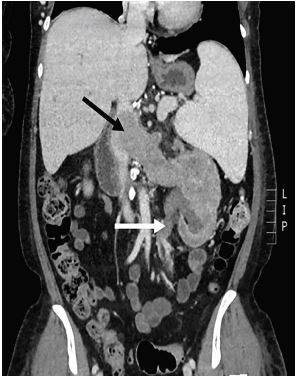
Coronal view of Computed Tomography of abdomen/pelvis with intravenous contrast demonstrating a necrotic mass in the left kidney with extension into the inferior vena cava (black arrow) and ureter (white arrow).
